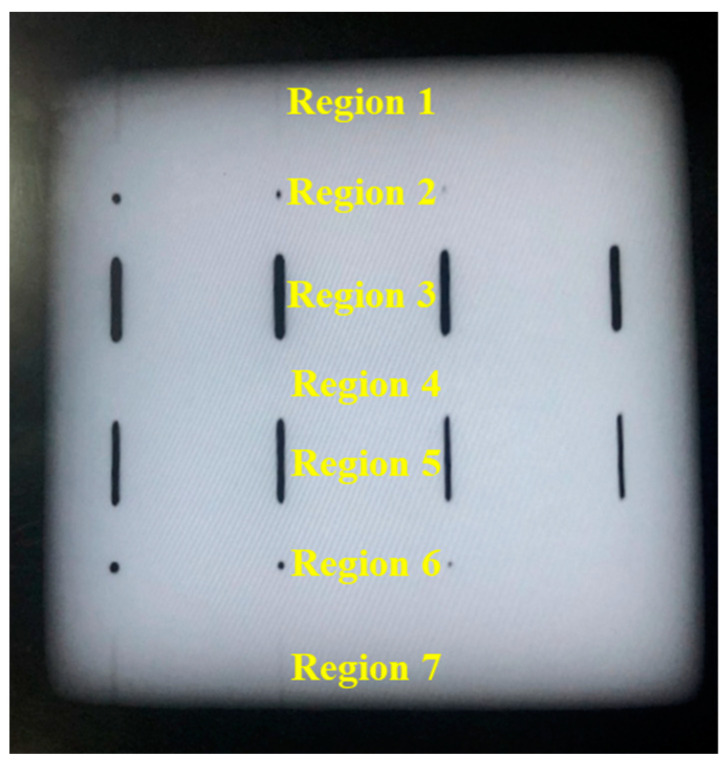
The result of the digital radiograph inspection.
